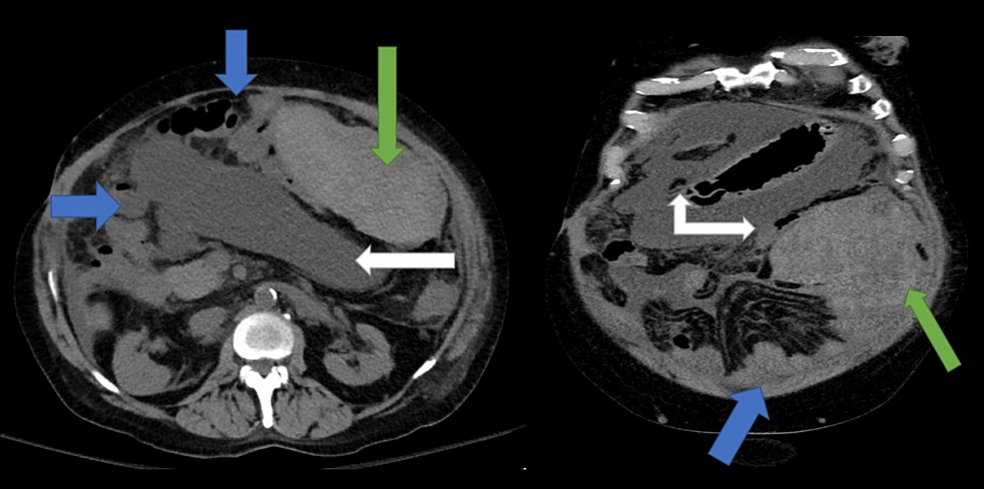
CT abdomen and pelvis Axial view (left) and coronal view (right) demonstrate a large peritoneal hematoma (green arrows), normal small bowel loops (blue arrows), and large ascites (white arrows) CT: computed tomography
